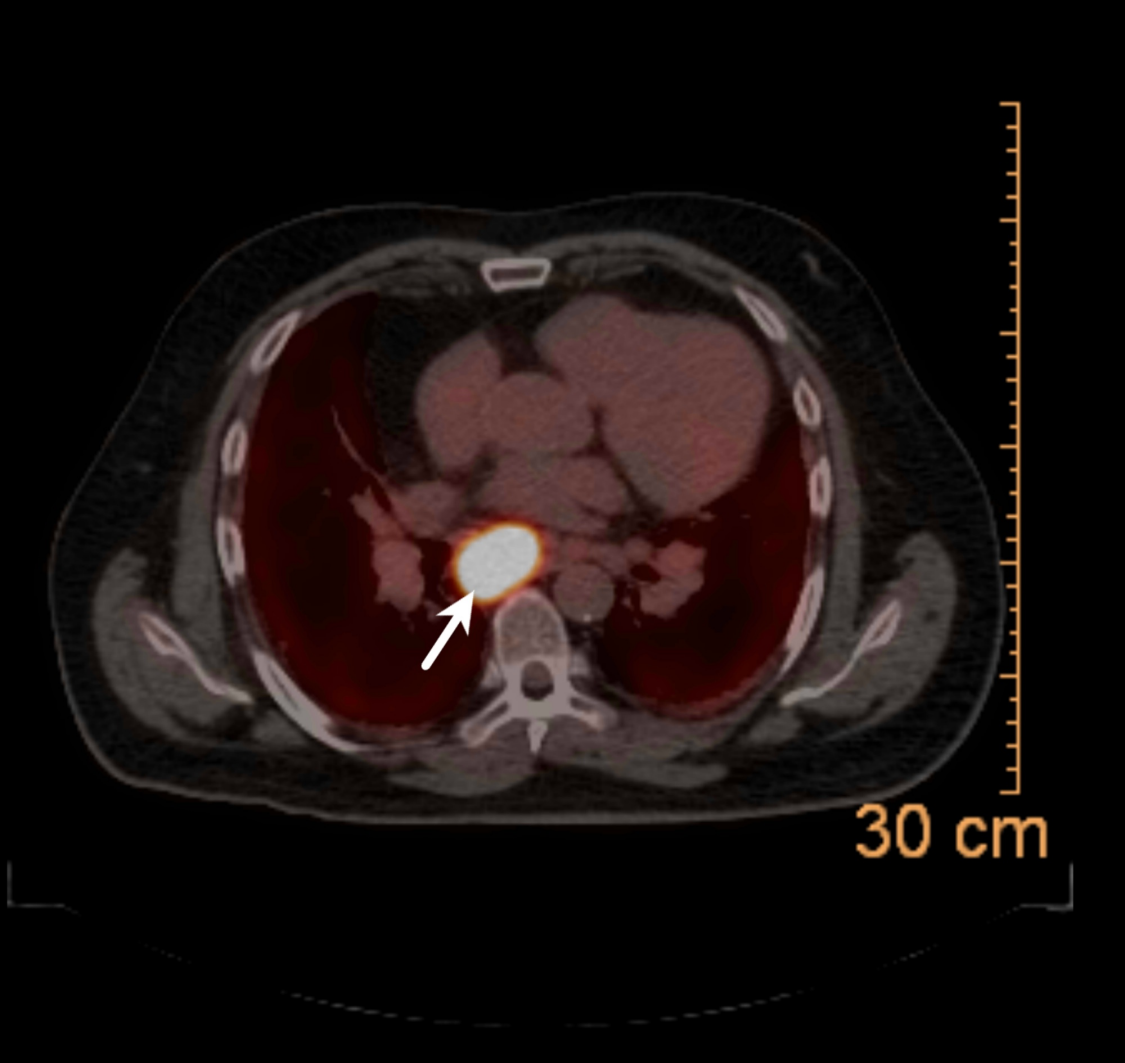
Positron emission tomography-CT showing avid lymph node in the subcarinal portion of the mediastinum (arrow)
